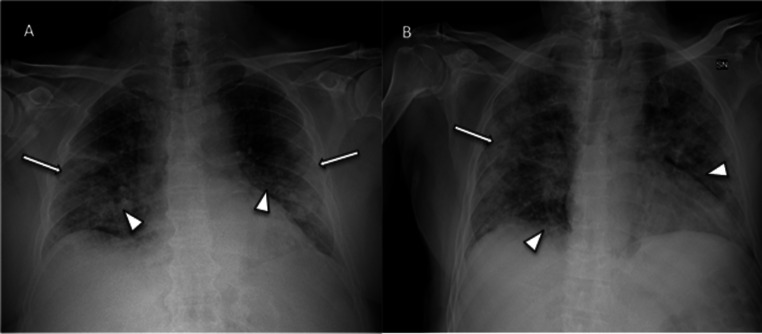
Chest radiography findings in two confirmed COVID-19 patients. CXRs in AP projection of two different patients (A, B) show subpleural ground-glass opacities (arrows) in external middle and lower lung areas and interstitial reticular pattern in internal areas (arrowheads). Both CXRs were judged as positive for COVID-19 by the expert reader and by the less expert readers; both patients had positive rRT-PCR results
